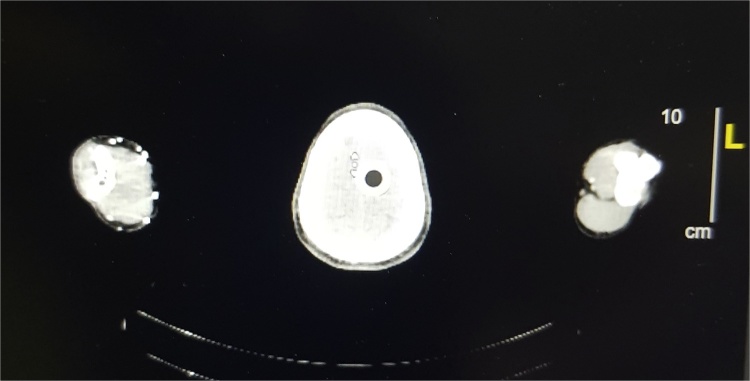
18 FDG PET-CT (Non-contrast) showing metabolically active left axillary lymph nodes.
